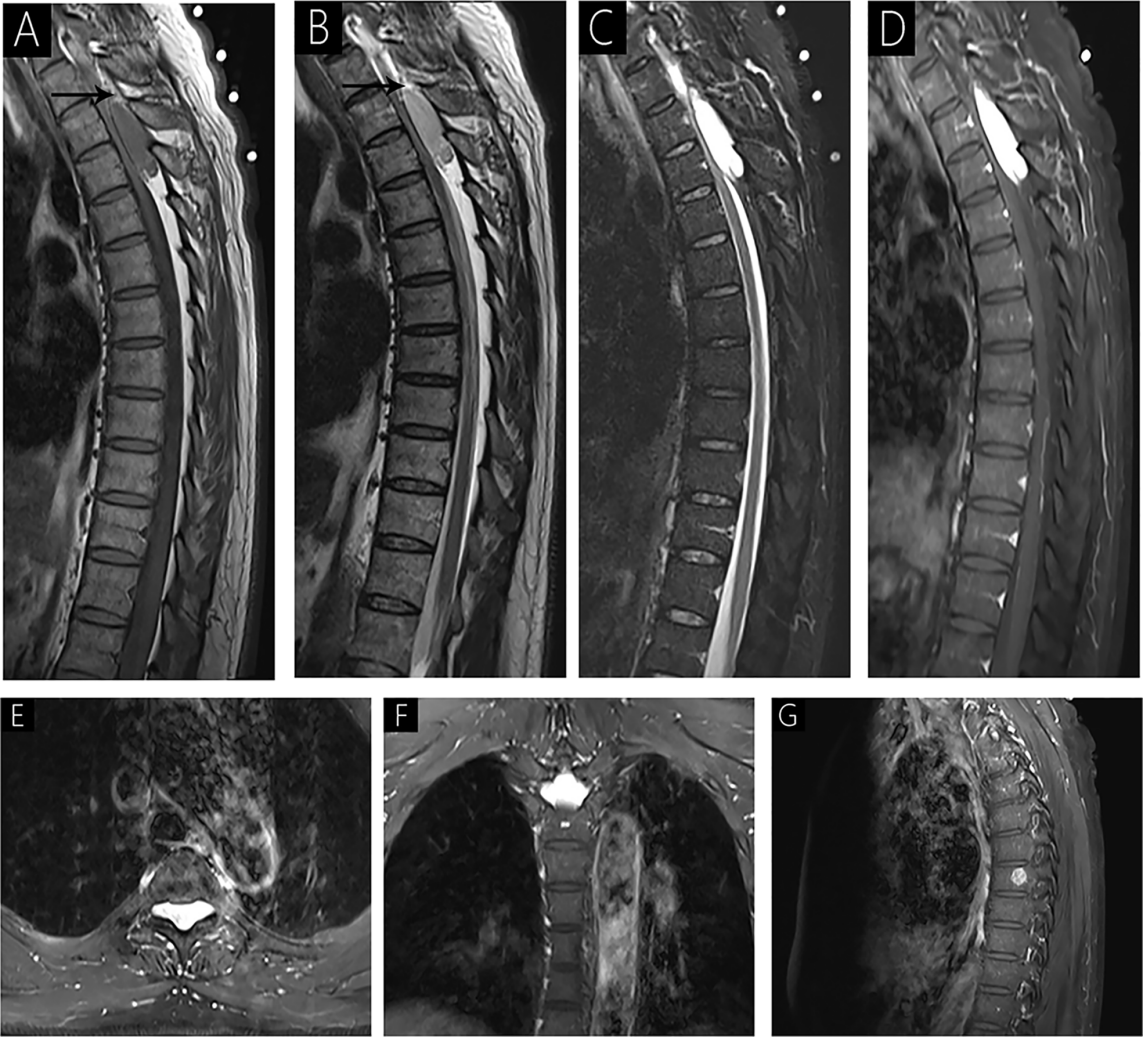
Case 1 (T3-T4) shows an isointense signal on T1WI (A); a mild hyperintense signal on T2WI (B); hyperintense signal on fat-saturated T2WI (C); and homogenous signal with significant enhancement on enhanced MRI (D–F). Sagittal T1WI and T2WI shows an epidural mass causing anterior compression of the thecal sac and peripheral fat tissues (arrow). The spinal cord is markedly compressed with striped slight hyperintensity internally on fat-saturated T2WI (C). Intervertebral foramen extension is noted on axial T1WI with contrast (E, F). A lesion is observed in the 8th vertebra with significant enhancement on sagittal T1WI with contrast (G).
